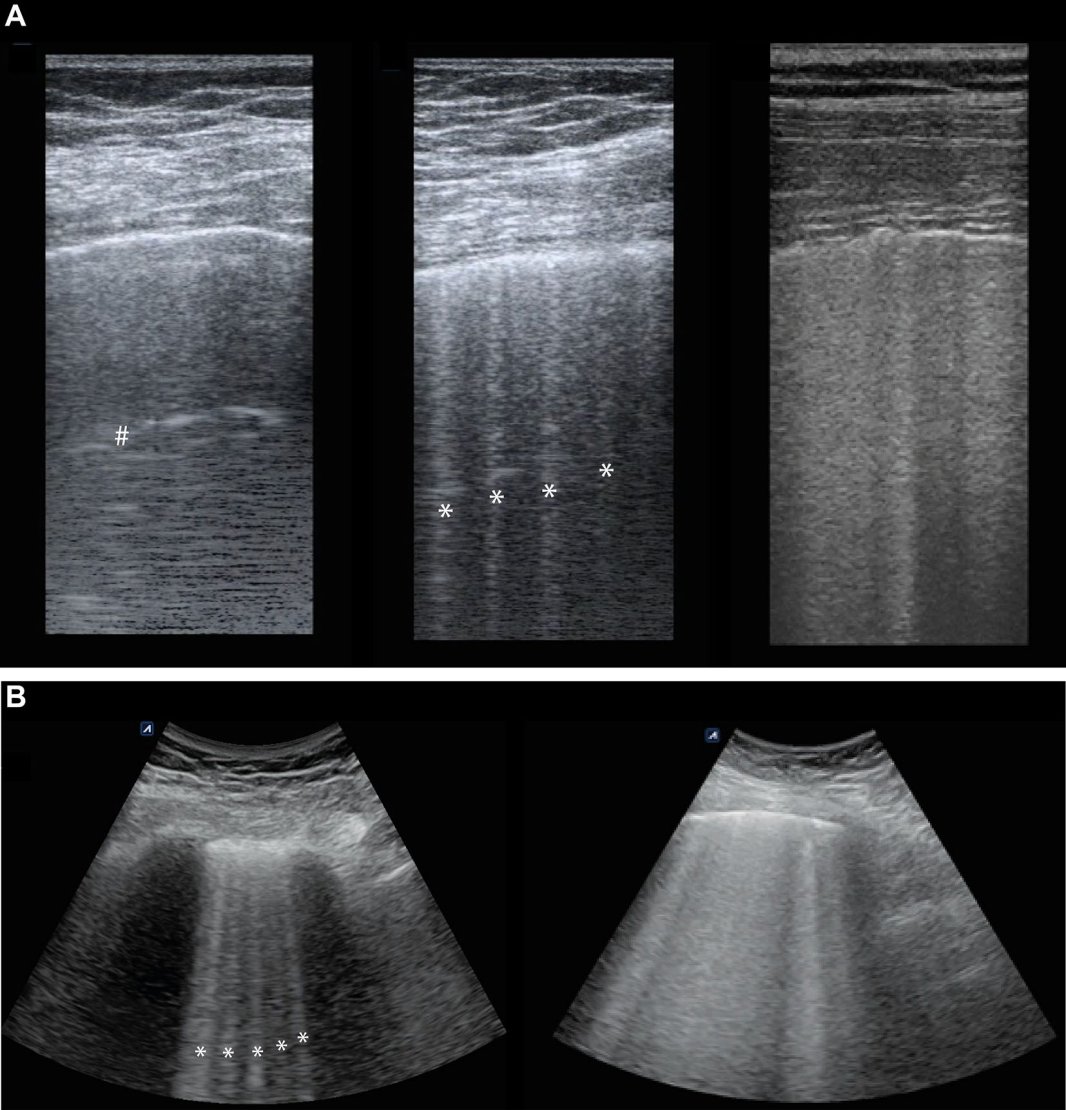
Lung ultrasound images used for aeration and B-line count scores. A: Example of lung ultrasound (LUS) images used for 8- and 12-region aeration scores using linear array probe (5.0–12.0 MHz). Left image: a line indicated by # (A pattern, 0 points); center image: ≥ 3 well-spaced B-lines indicated by * (B1 pattern, 1 point) with thickened pleura; right image: coalescent B-lines (B2 pattern, 2 points) with irregular, fragmented pleura. B: example of LUS images used for the B-line score using curved array probe (2.5–5.0 MHz). Left image: five B-lines, resulting in 5 points; right image: confluent B-lines taking up 100% of the intercostal space, resulting in 8 points. LUS  lung ultrasound
